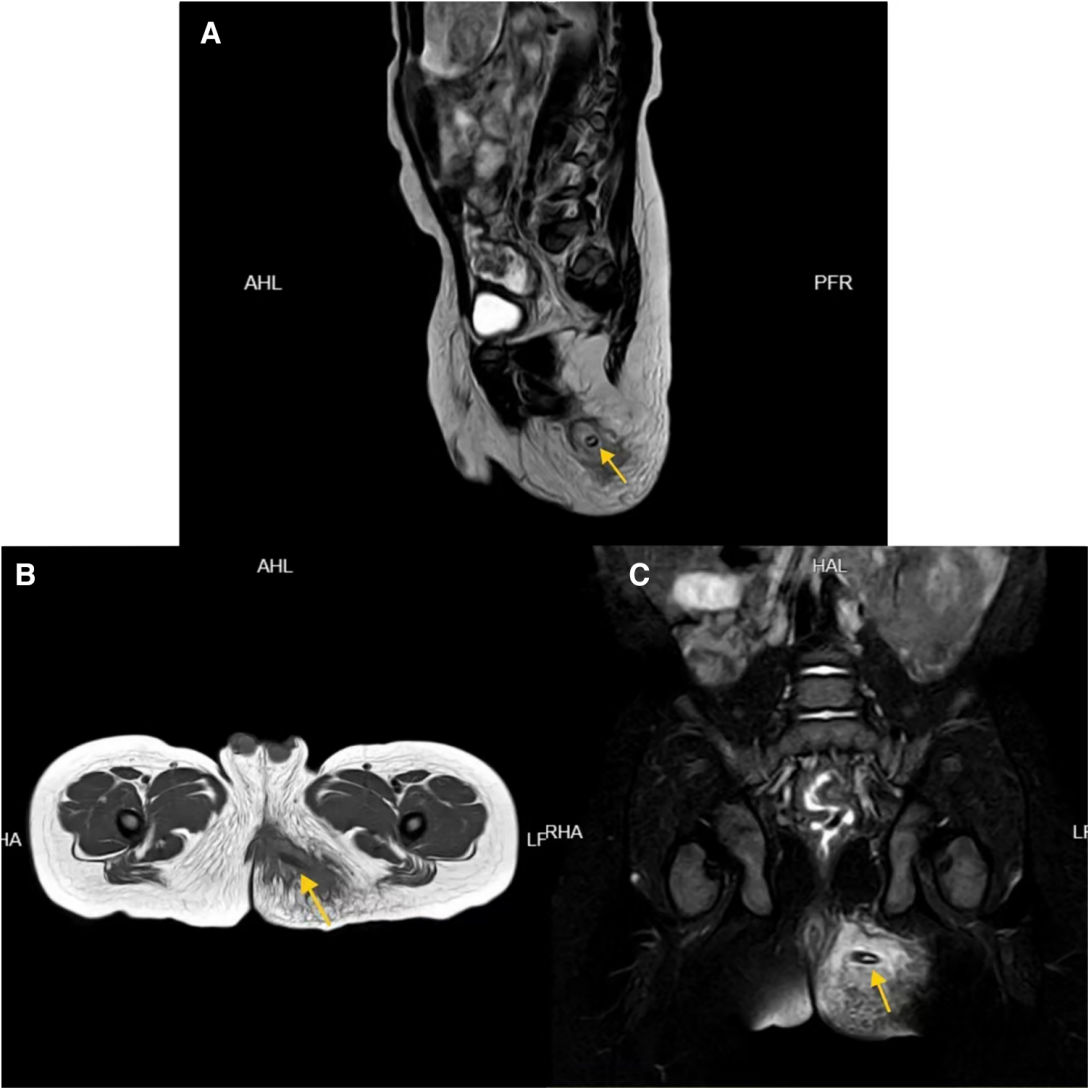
MRI images of patient 1 in the sagittal (A), horizontal (B), and coronal plane (C) showing the jujube pit (indicated by arrows).
